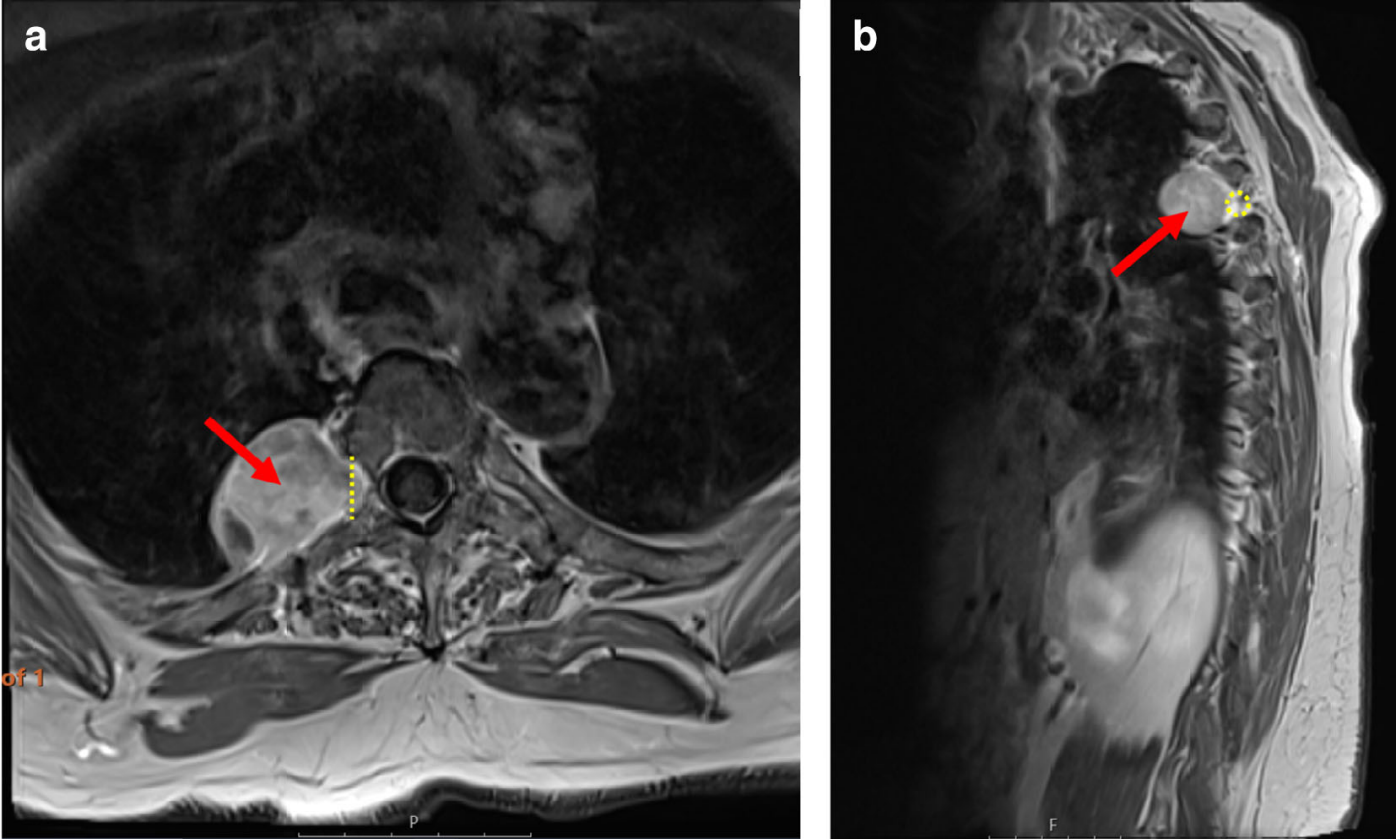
Preoperative T1 weighted magnetic resonance imaging (MRI) of Patient 3 demonstrating a paraspinal dumbbell tumor in the right hemithorax at the T4/5 level (red arrow) extending into the intervertebral foramen (yellow dotted line and circle). (a) axial section; (b) sagittal section
